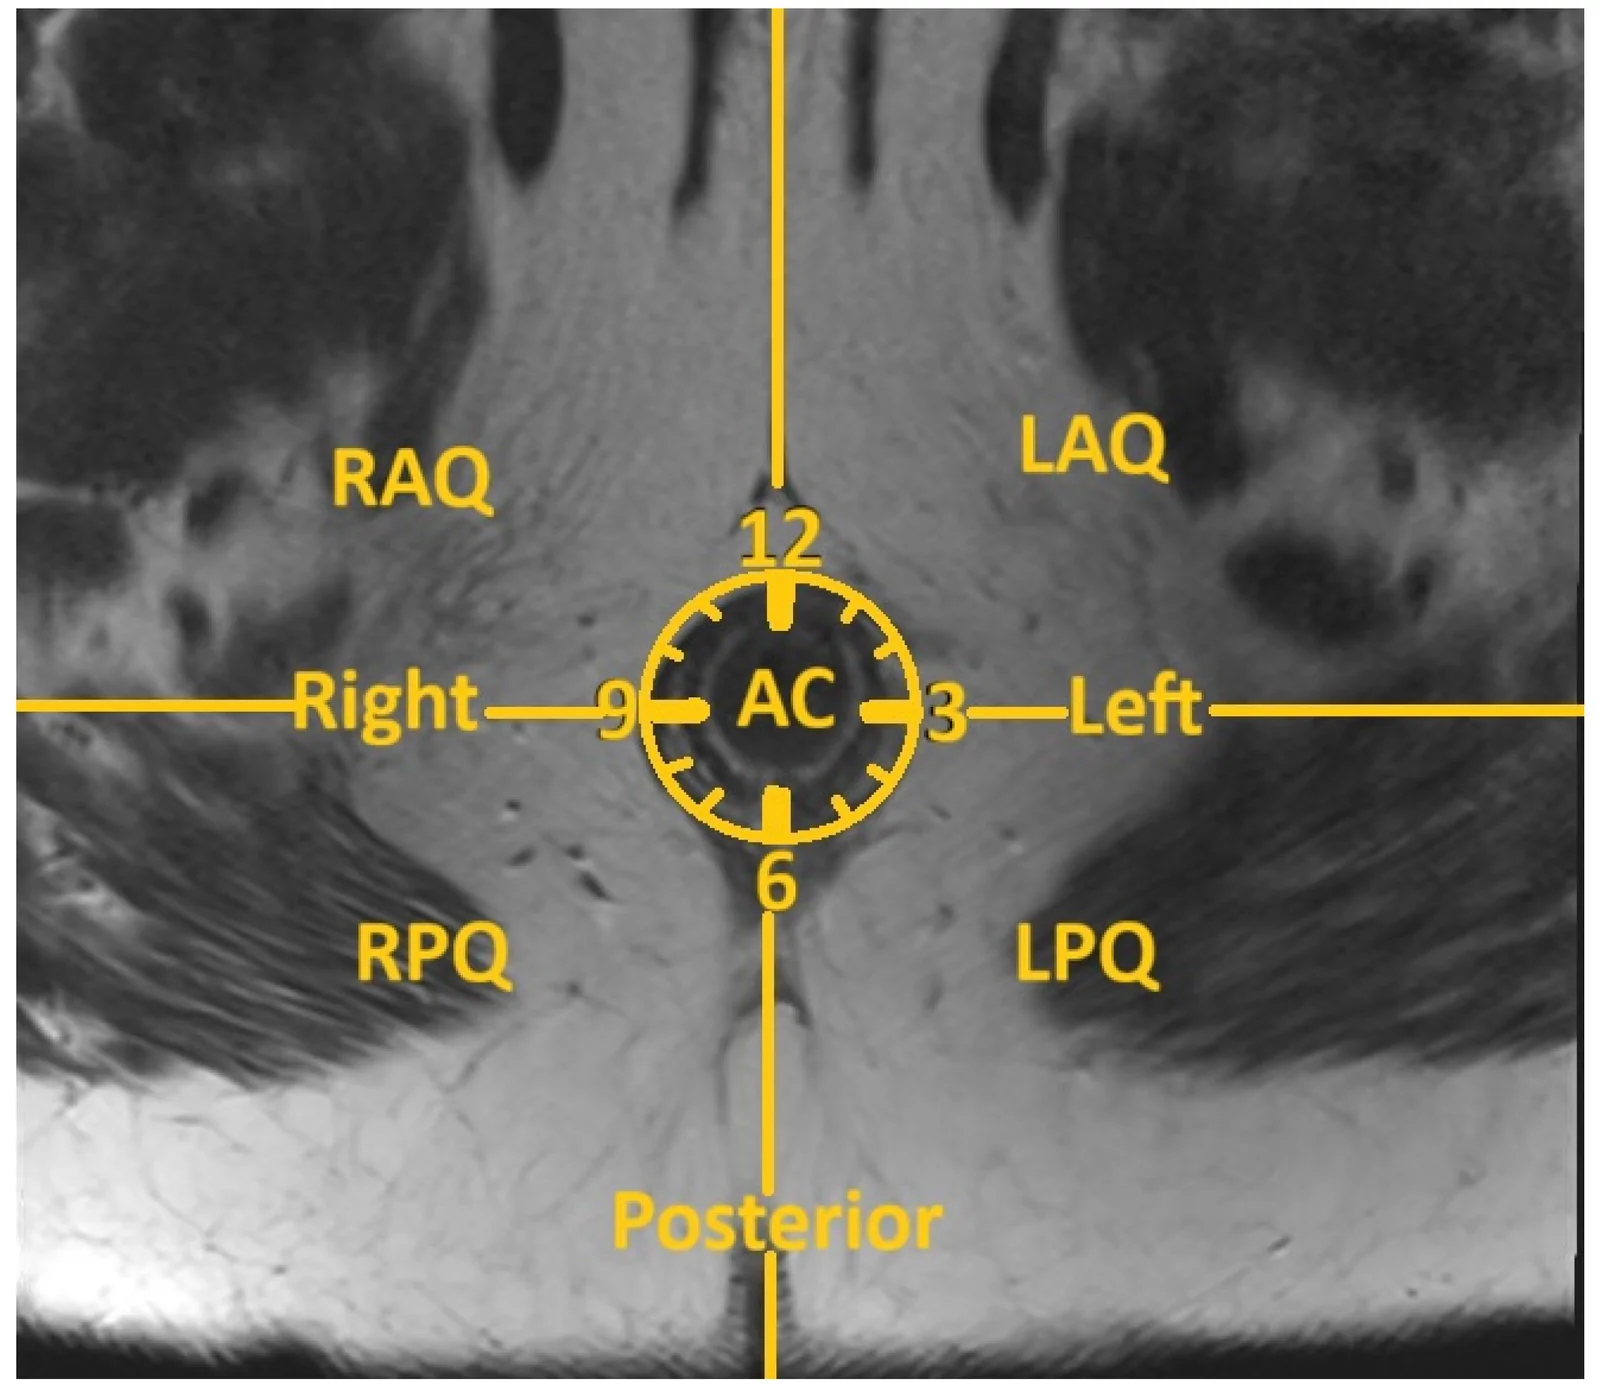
Axial magnetic resonance image of the perianal region in the lithotomy position, dividing the clockwise orientation of the anal canal into four quadrants and the 12 o’clock position. LAQ: Left anterior quadrant; LPQ: Left posterior quadrant; RPQ: Right posterior quadrant; RAQ: Right anterior quadrant; AC: Anal canal.
